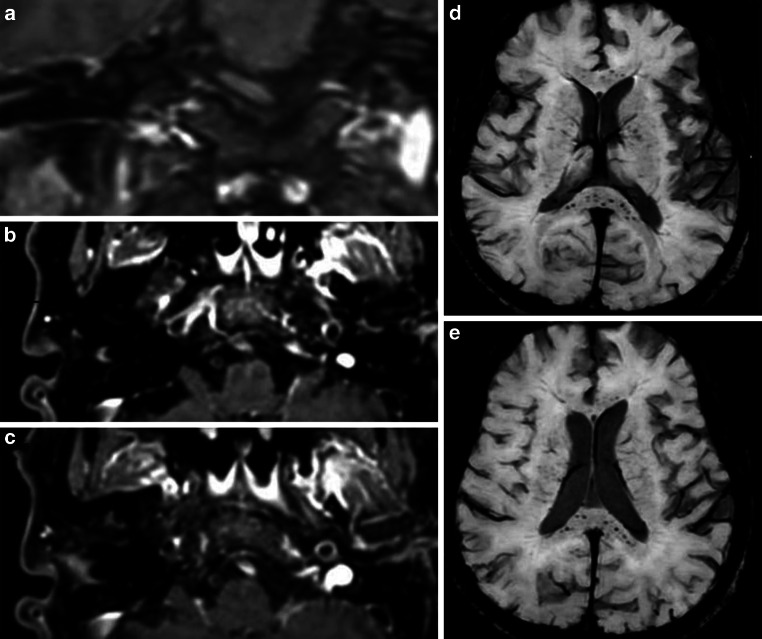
A 62-year-old patient hospitalized for acute respiratory distress syndrome due to SARS-CoV‑2 infection with intubation over 13 days. This patient was overweight and had mild cognitive impairment. He had developed a persistent delirium over more than 10 days after withdrawal of all drugs and without focal neurological deficit. MRI shows strong enhancement of the left vertebral artery (a–c), SWI images show microbleeds, preferentially in the corpus callosum (d, e)
